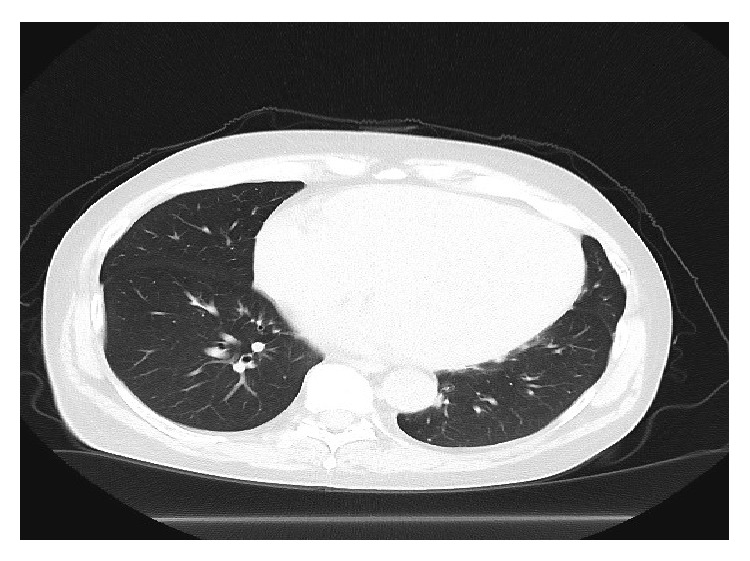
CT scan 12 months after stopping Vemurafenib.
